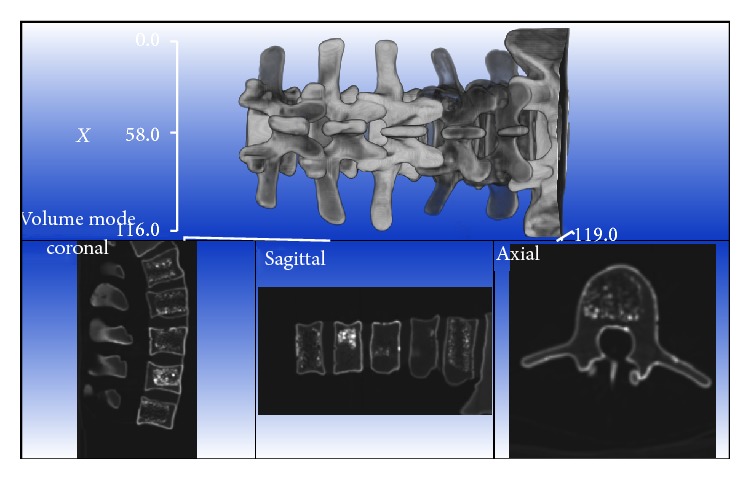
A reconstructed 3D CT spine model with axial, coronal, and sagittal views.
